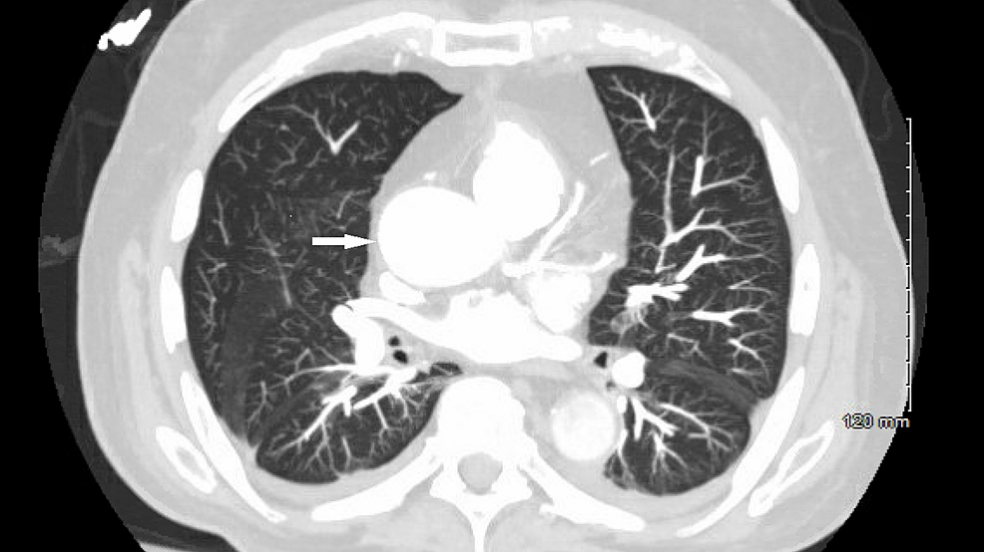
Chest CT (axial view) showing ascending aortic dilation (white arrow).
